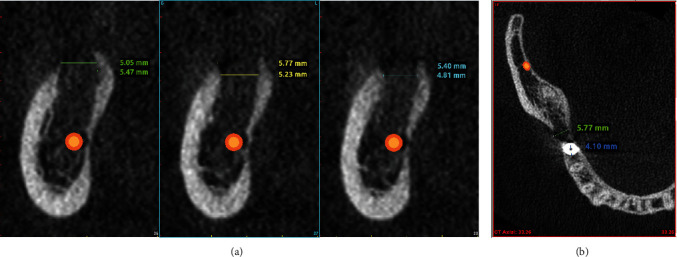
CBCT data after implant displacement. (a) (left) Vertical cuts. (b) (right) Axial reslice. Measurements of the implant bed at the site of displaced implant. The data show that the osteotomy dimensions are significantly larger than the diameter of the implant used. The lower border could not be determined.
